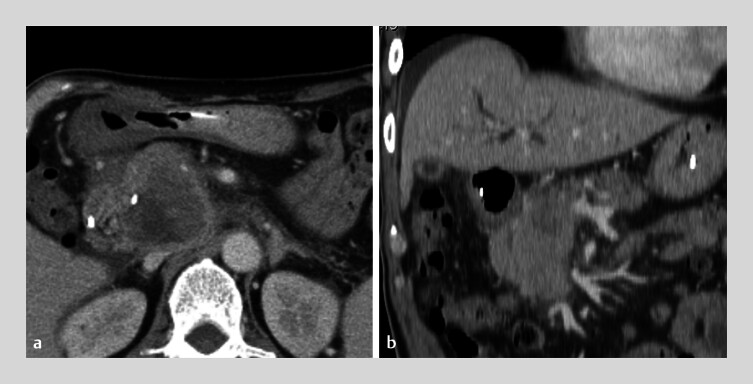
Computed tomography images showing obstructive jaundice due to distal biliary cancer.
